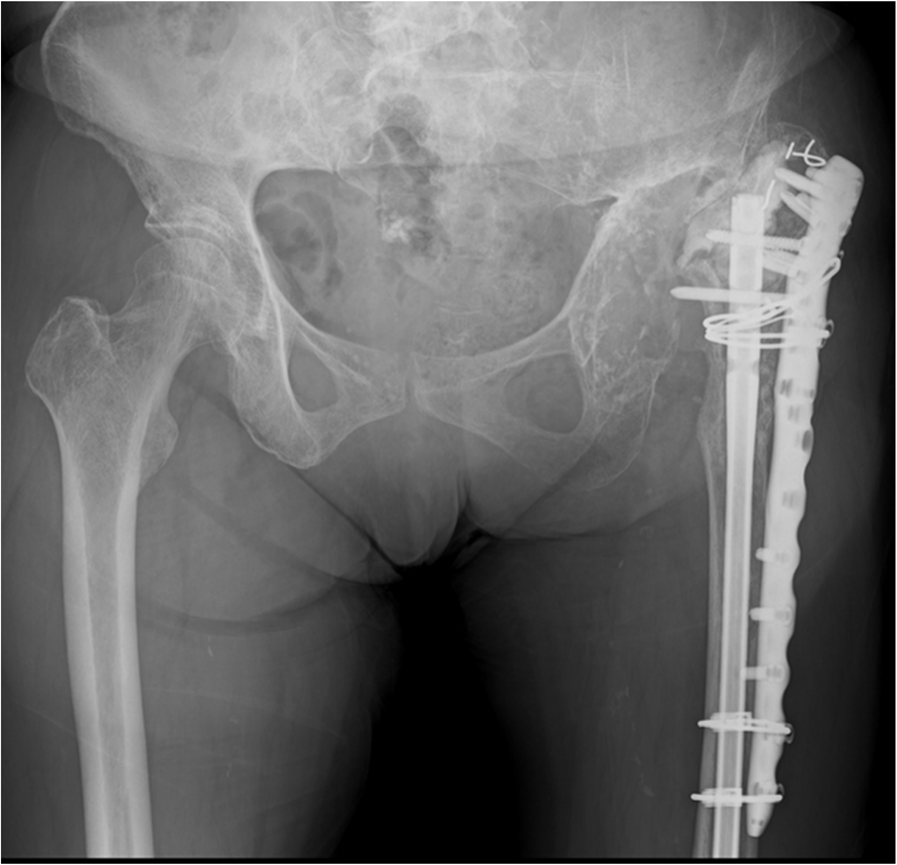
A plain anteroposterior radiograph of the bilateral hip 2 years and 9 months after the second surgery. Bone union can be observed
